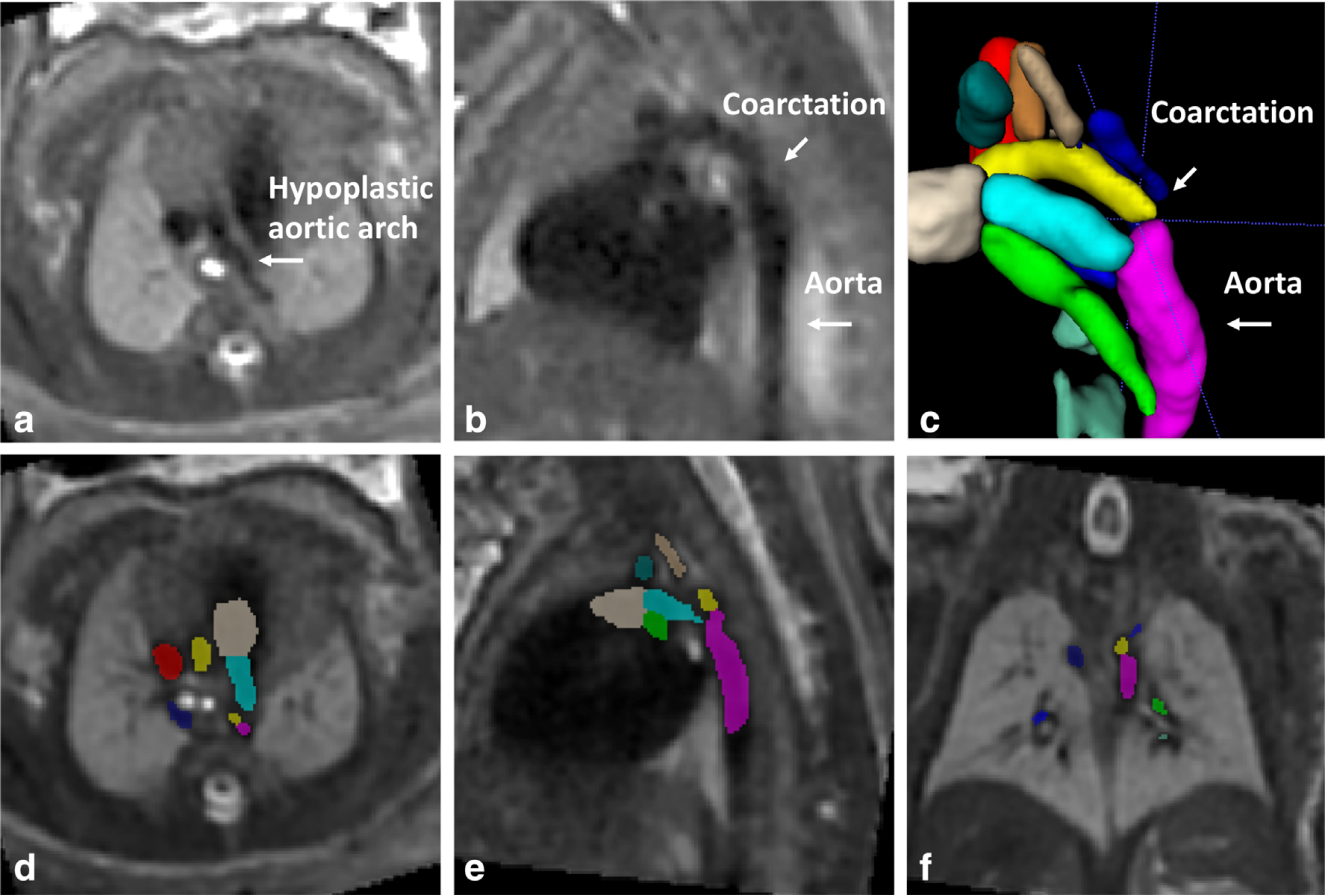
Fetal cardiovascular MRI in a fetus GA 34+ weeks with hypoplastic left heart syndrome obtained at 3.0 T MRI. Presented are: (a) a hypoplastic aortic arch and (b) aortic coarctation with poststenotic dilatation of the descending aorta. (c) Shows the aortic coarctation in the 3D reconstruction, which is based on vessel segmentation presented in (d–f). Movie S4a,b in the Supplemental Material show the excellent image quality for vascular (venous) assessment.
